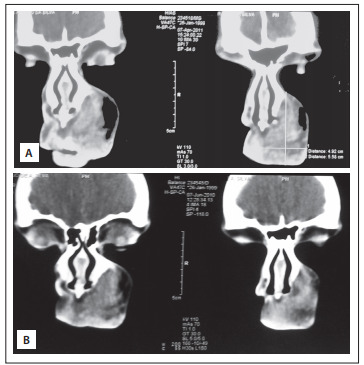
Computed tomography scans on a patient with a congenital facial lesion, contrast enhancing, non-involutive. Imaging and clinical history were used for diagnosing non-involutive congenital hemangioma (NICH). Propranolol treatment did not change the estimated lesion size. Upper panel (A) shows lesion after the treatment, whereas lower panel (B) shows the lesion 10 months earlier.
